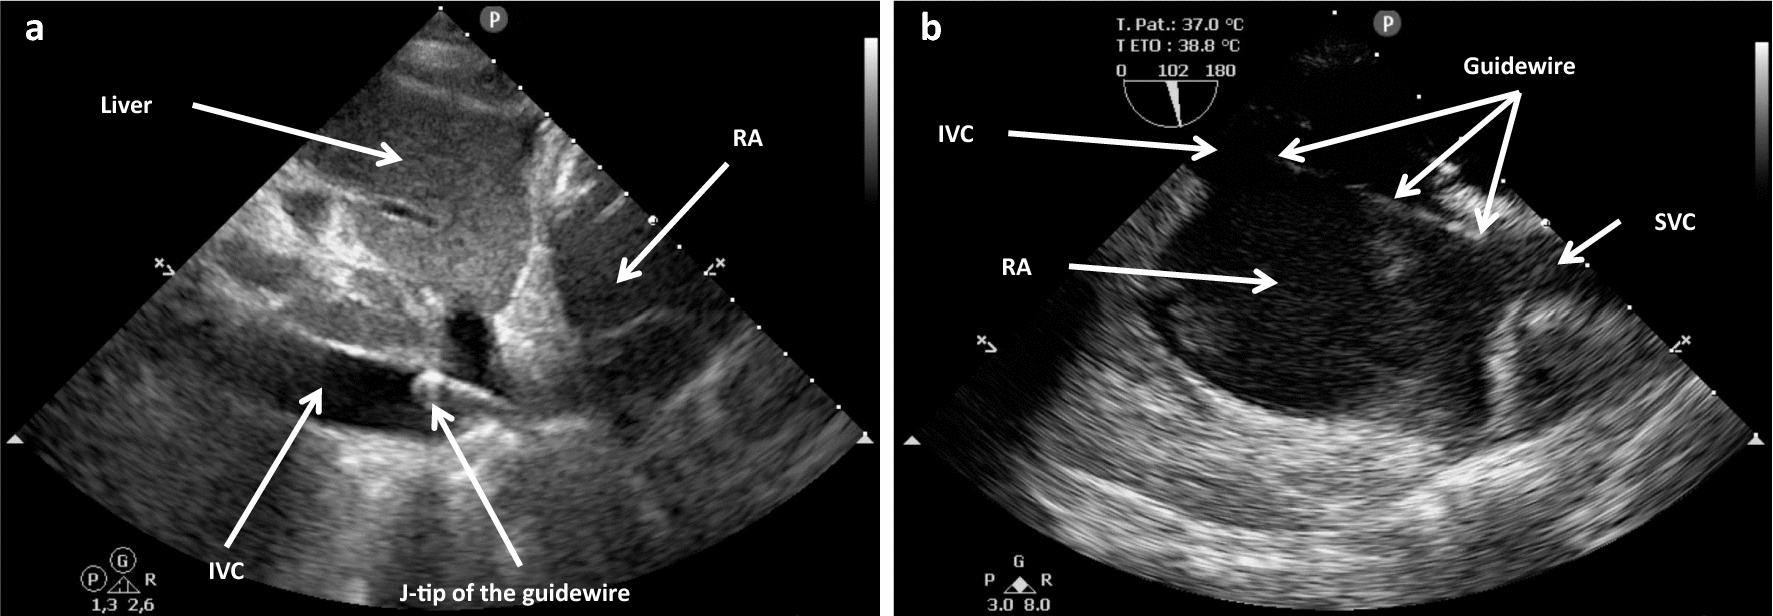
a Transthoracic echocardiography subcostal view showing the J-tip of the guidewire entering the inferior vena cava. b Transesophageal echocardiography bicaval view showing the guidewire passing through from the superior vena cava into the right atrium and entering into the inferior vena cava. *RA* right atrium, *SVC* superior vena cava, *IVC* inferior vena cava
